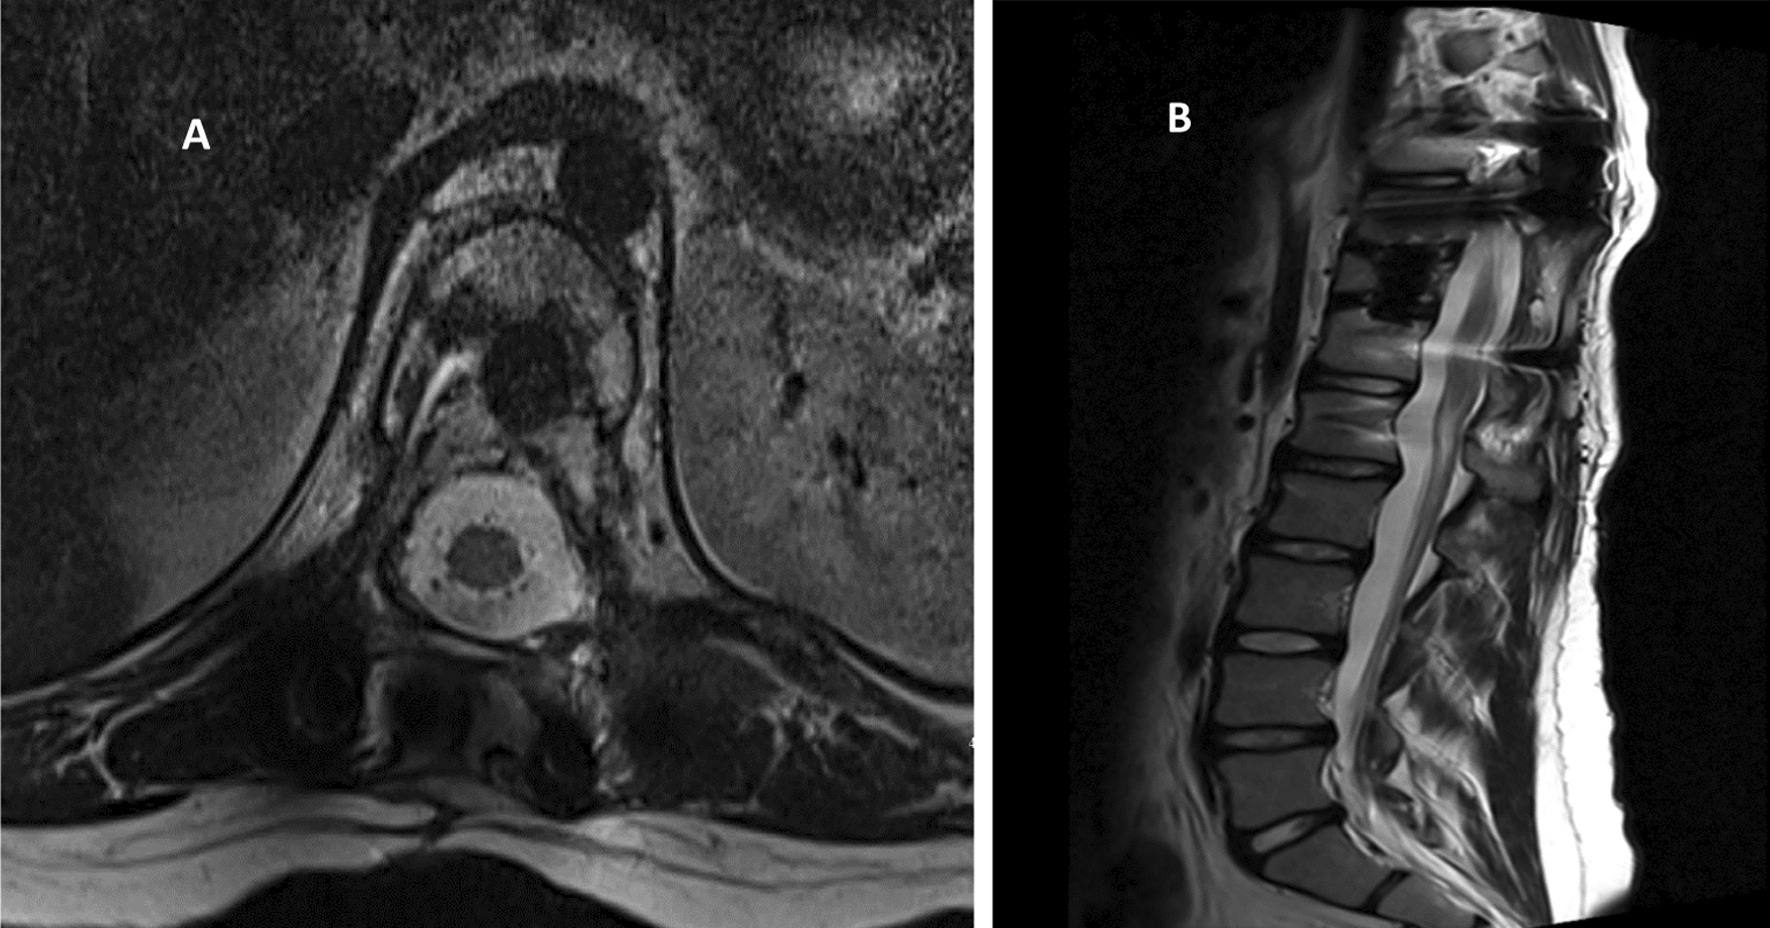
A, B MRI A axial and B sagittal showing partially resected T11 with presence of a vertical mesh cage in place with no evidence of residual or recurrent aneurysmal bone cysts
